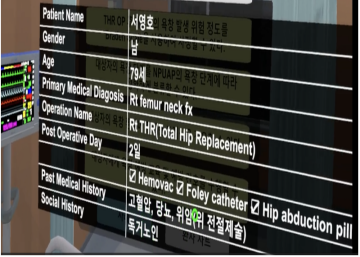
Patient information			Identifying data: vital signs, results of blood test, x-ray findings, physical exam, medication
